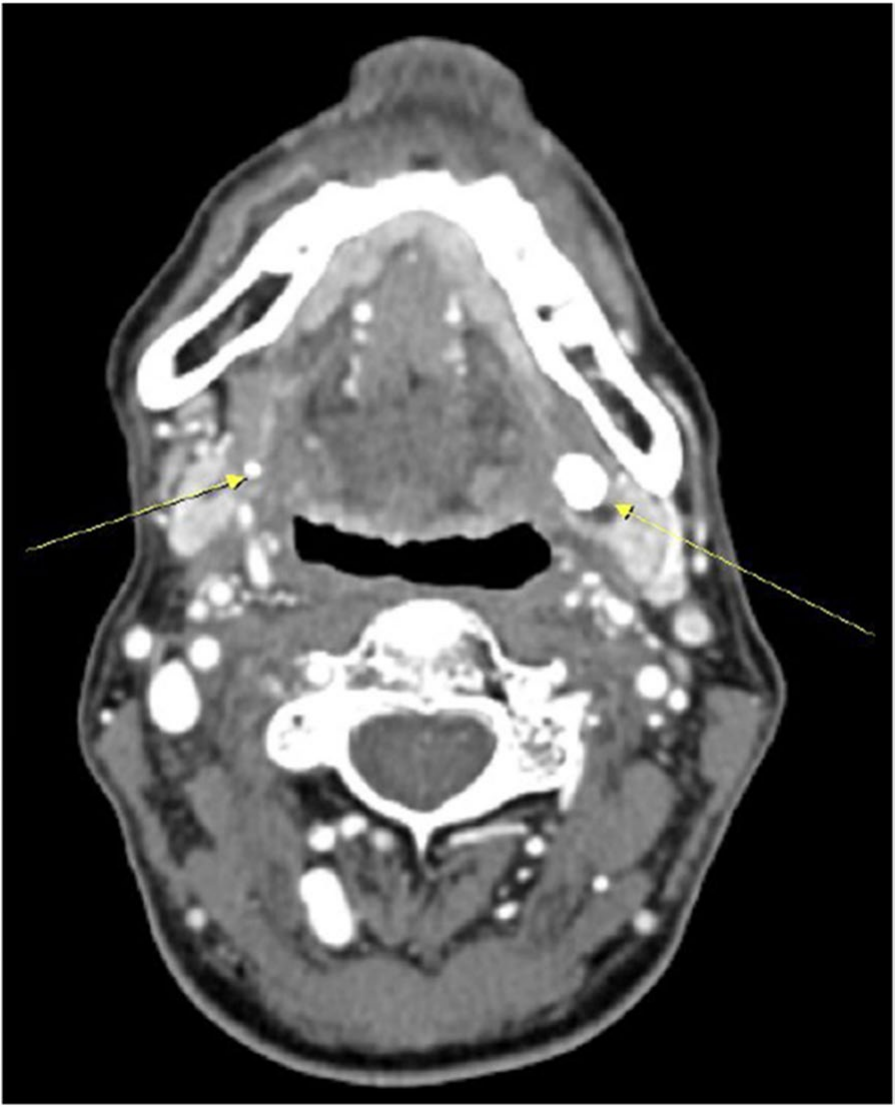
Neck CT (enhanced) image with sialolithiasis
